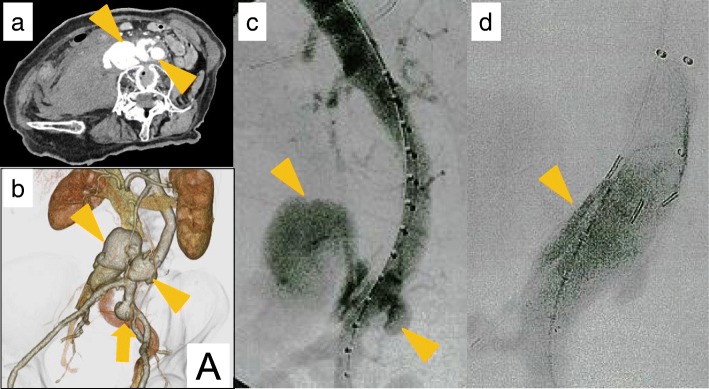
Images of the abdominal aortic aneurysm before and during the primary operation. a A computed tomography (CT) scan of the abdomen and pelvis demonstrated rupture of the abdominal aortic aneurysm (orange arrow head). b A 3D-CT scan reconstruction of the abdomen and pelvis demonstrated the abdominal aortic aneurysm with a fistulous communication between the aneurysm and the inferior vena cava (orange arrowhead) and the left common iliac artery aneurysm (orange arrow). c Arterial angiography demonstrated the abdominal aortic aneurysm with fistulous communication between the aneurysm and the inferior vena cava (orange arrowhead). The location of aorta rupture was located 7.5 cm distal to the renal arteries and 2.5 cm proximal to the bifurcation of the aorta. d We deployed an ENDURANTII iliac extension far proximal to the terminal aorta that was long enough to place three or more stents (orange arrowhead)
